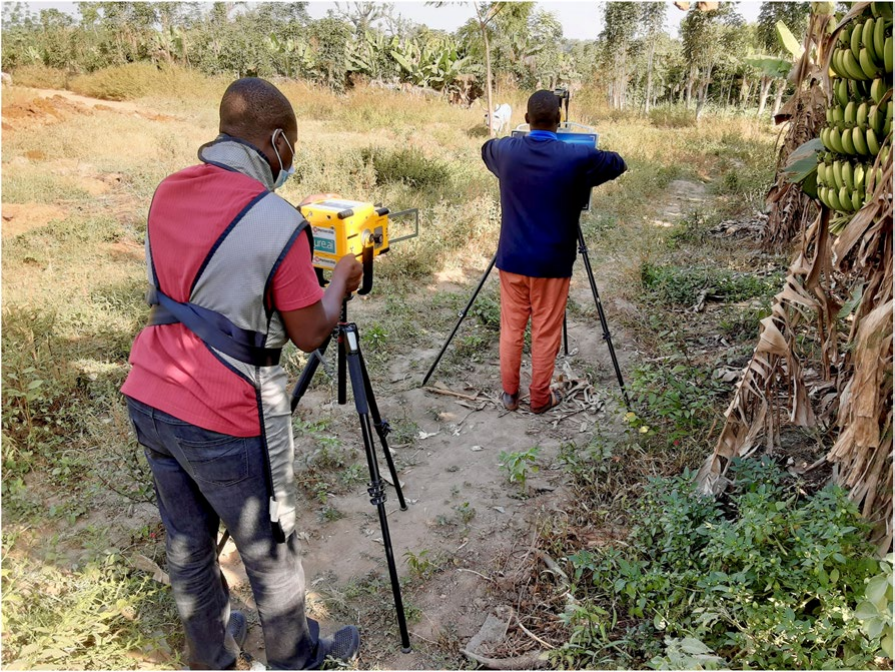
A demonstration of the capabilities of ultra-portable X-ray machines outside healthcare facilities
